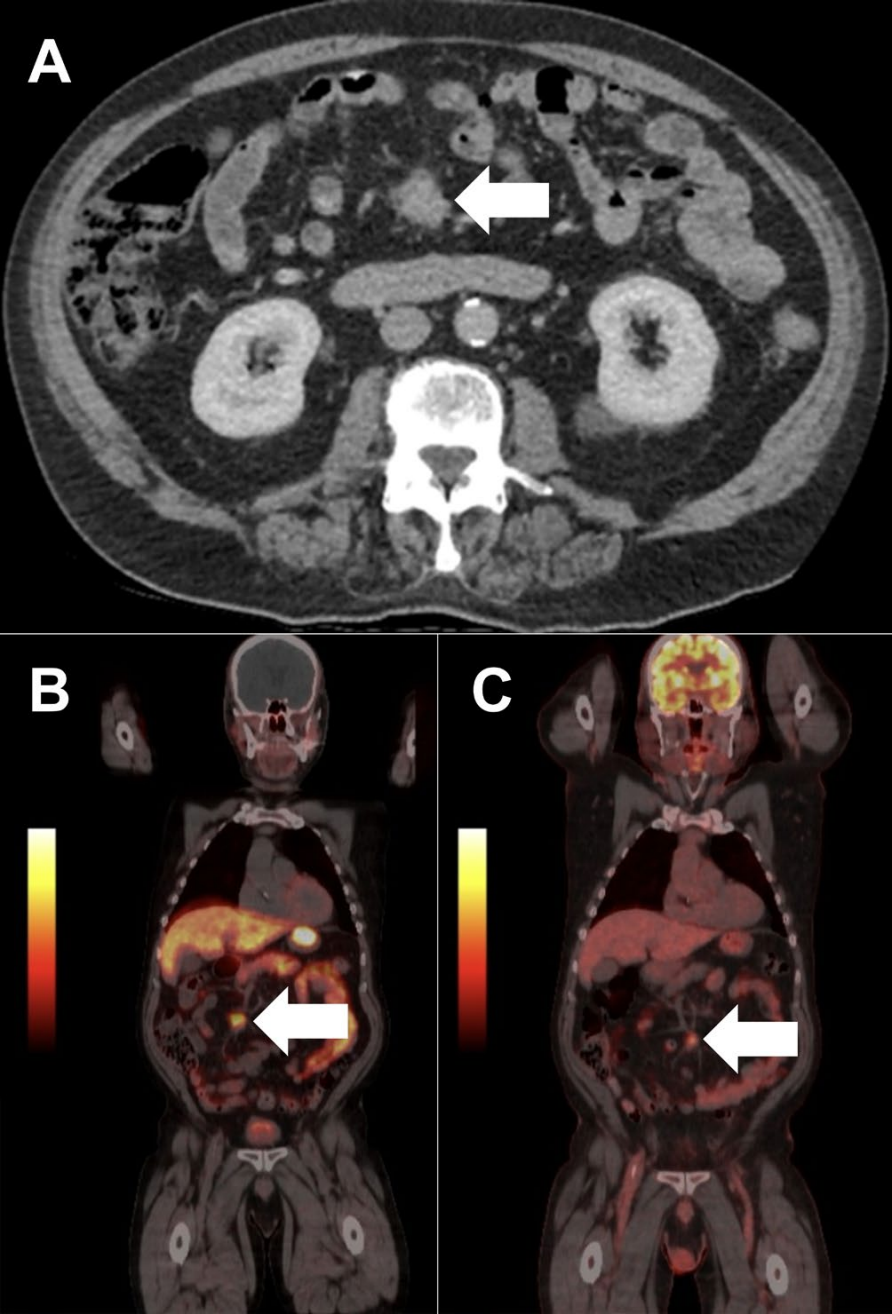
(A) Computed tomography (CT), (B) ^68Ga‐DOTATATE positron emission tomography (PET), and (C) FDG‐PET demonstrating an avid mesenteric deposit (white arrows), in the absence of a clear primary lesion.
